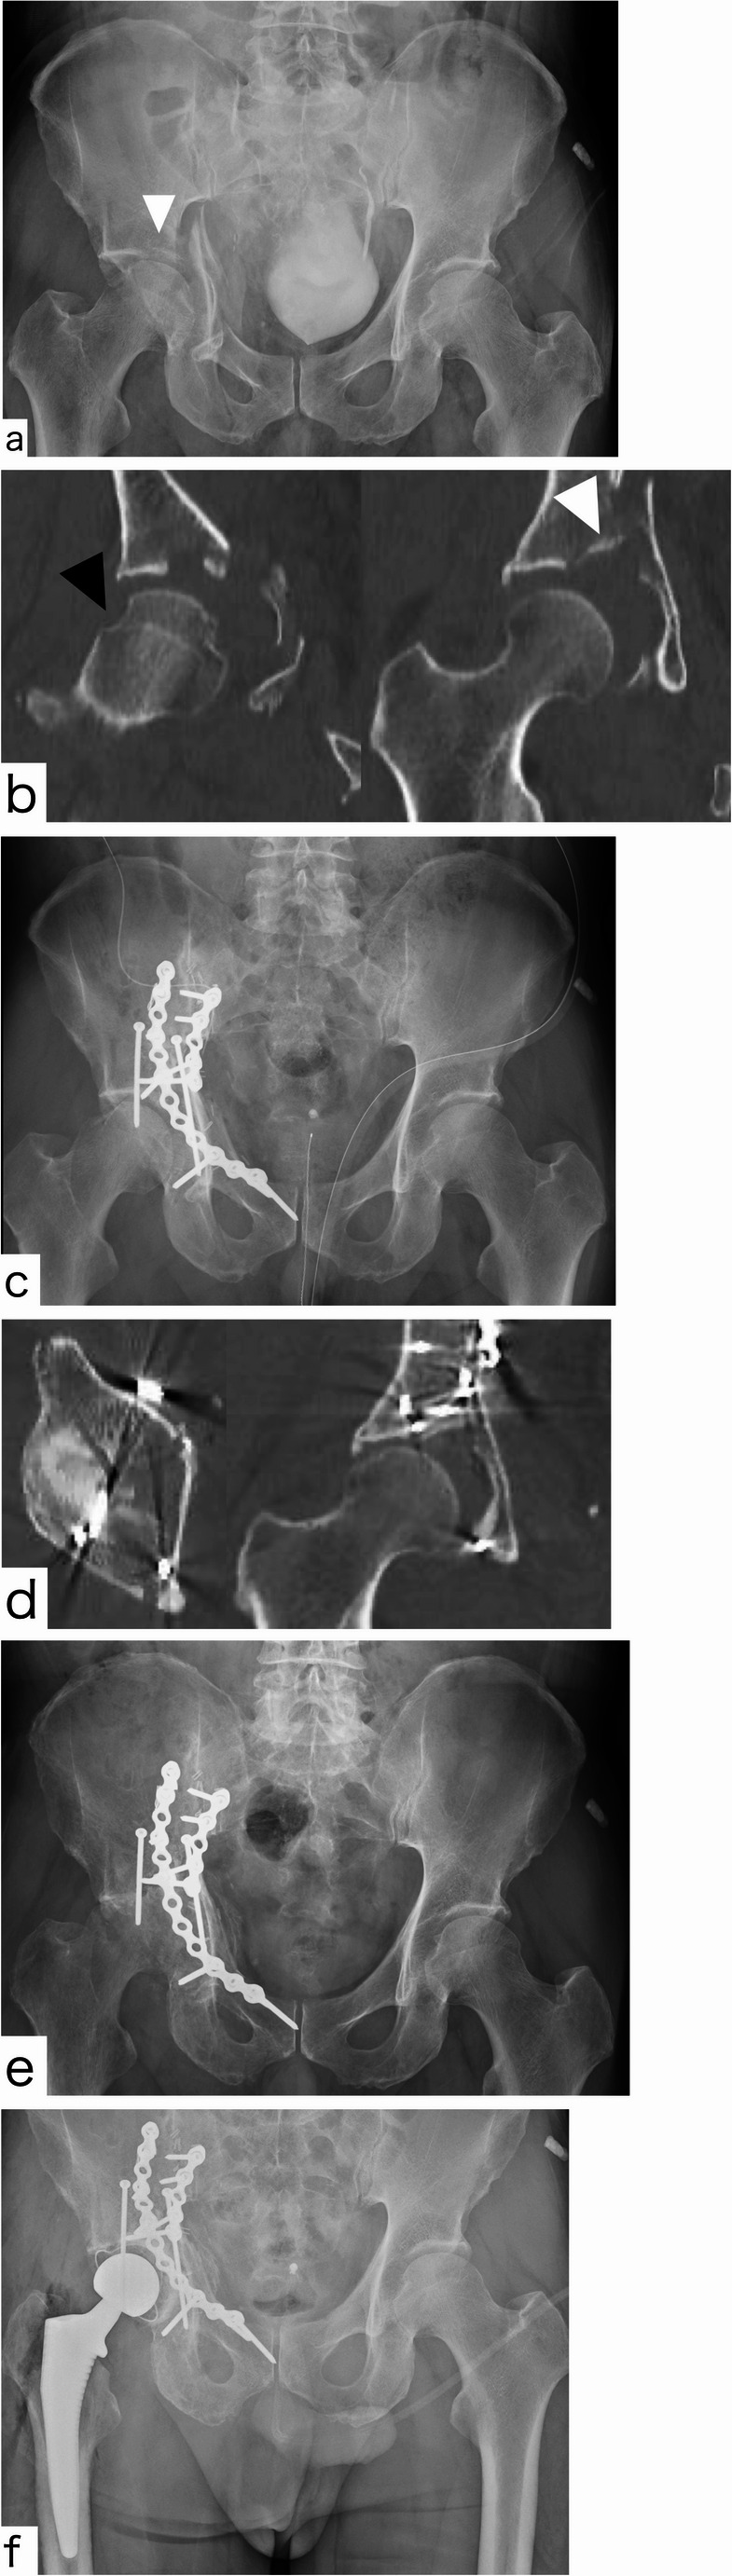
65-year-old male with anterior column and posterior hemitransvers fracture treated by ORIF using pararectus approach AP X-ray at the time of injury. Dome impaction and medialization of femoral head existed CT coronal views revealed femoral head impaction (black arrow) and dome impaction (white arrow) Postoperative AP X-ray Postoperative CT revealed poor reduction quality of impacted fragment AP X-ray at three months postoperatively shows end-stage hip arthritis. HHS at 3 months was 29.7 AP X-ray after the THA conversion
